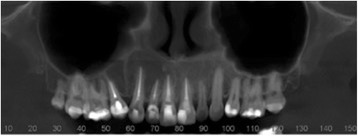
Panoramic image of computed tomography scan showing formation of a bony septum indicating new bone formation
